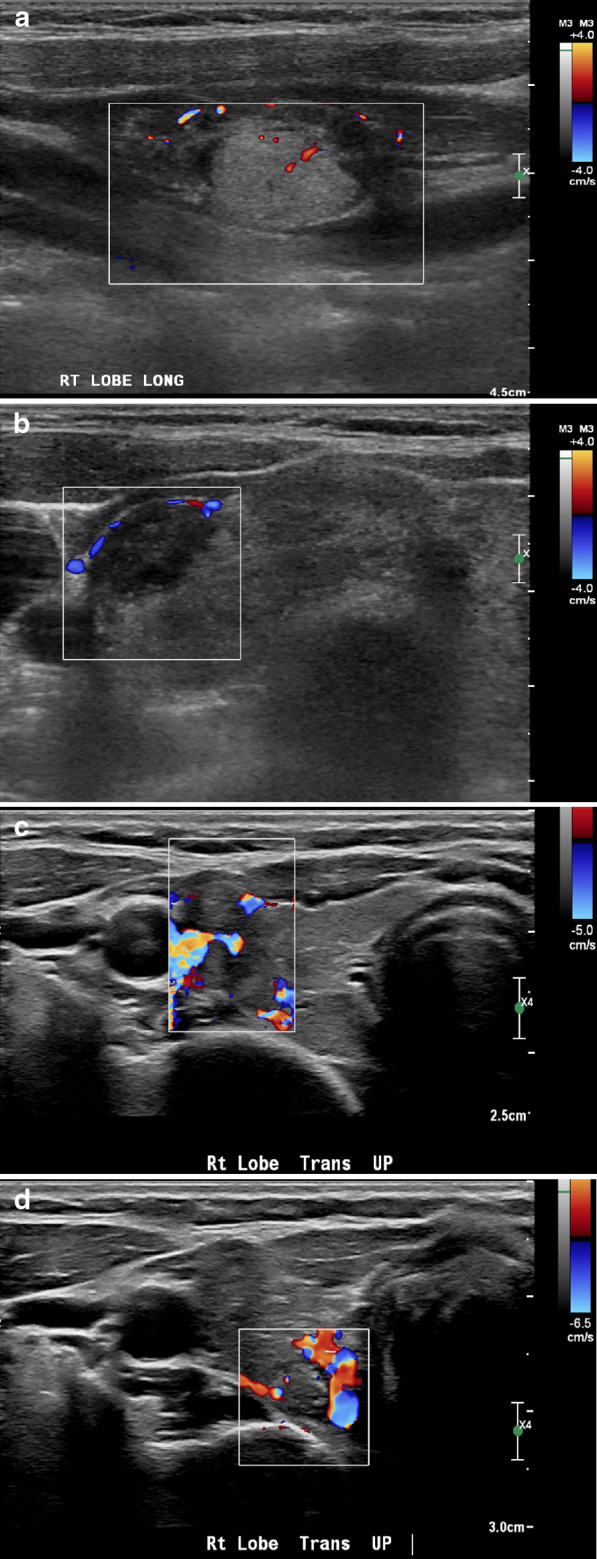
Multiple transverse and longitudinal Doppler ultrasound images performed by linear probe showing: a A hyperechoic nodule with internal vascularity seen within. b A hypoechoic nodule with peripheral vascularity seen surrounding it. c A non-homogenous isoechoic nodule with mixed peripheral and central vascularity. d A hyperechoic nodule with mixed peripheral and internal vascularity
